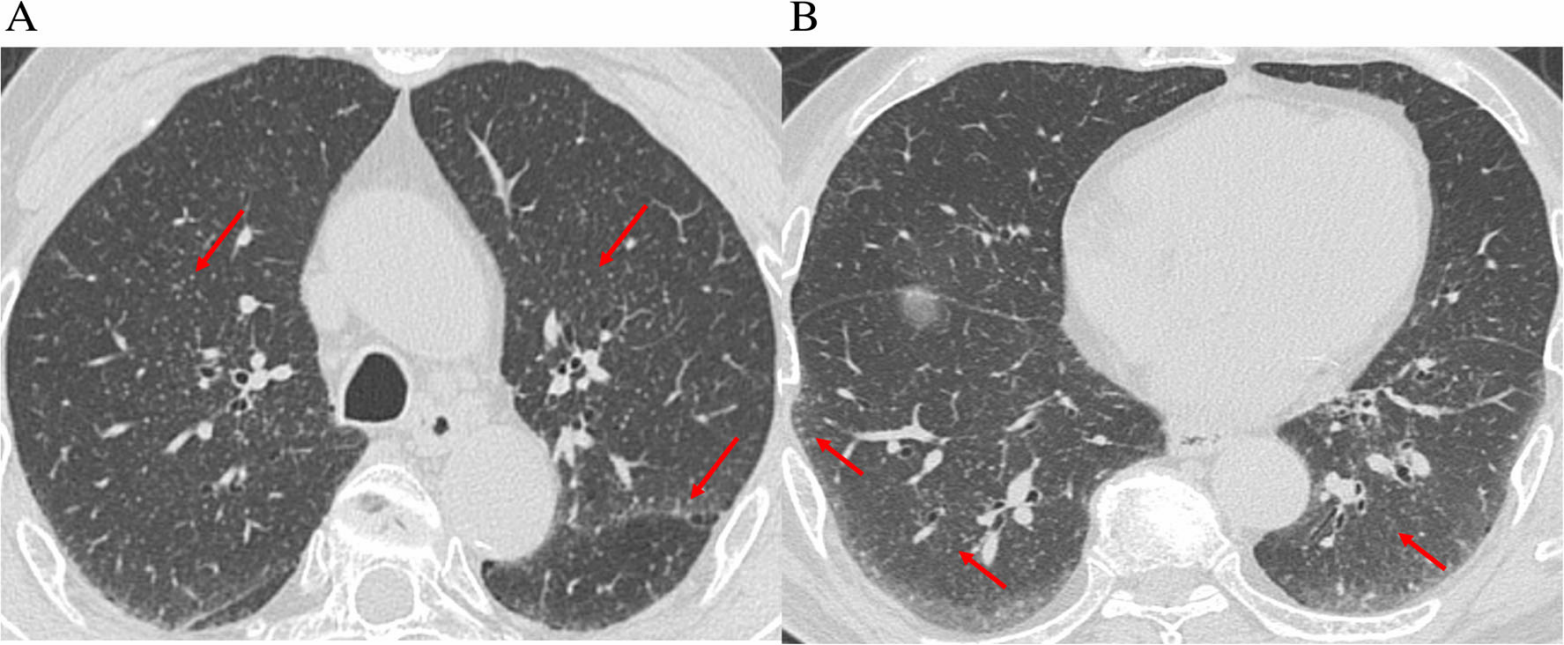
Chest computed tomography (CT). (A, B) CT reveals diffuse granular shadow along with the interlobular pleura and bronchial vessel bundles in the lung fields.
